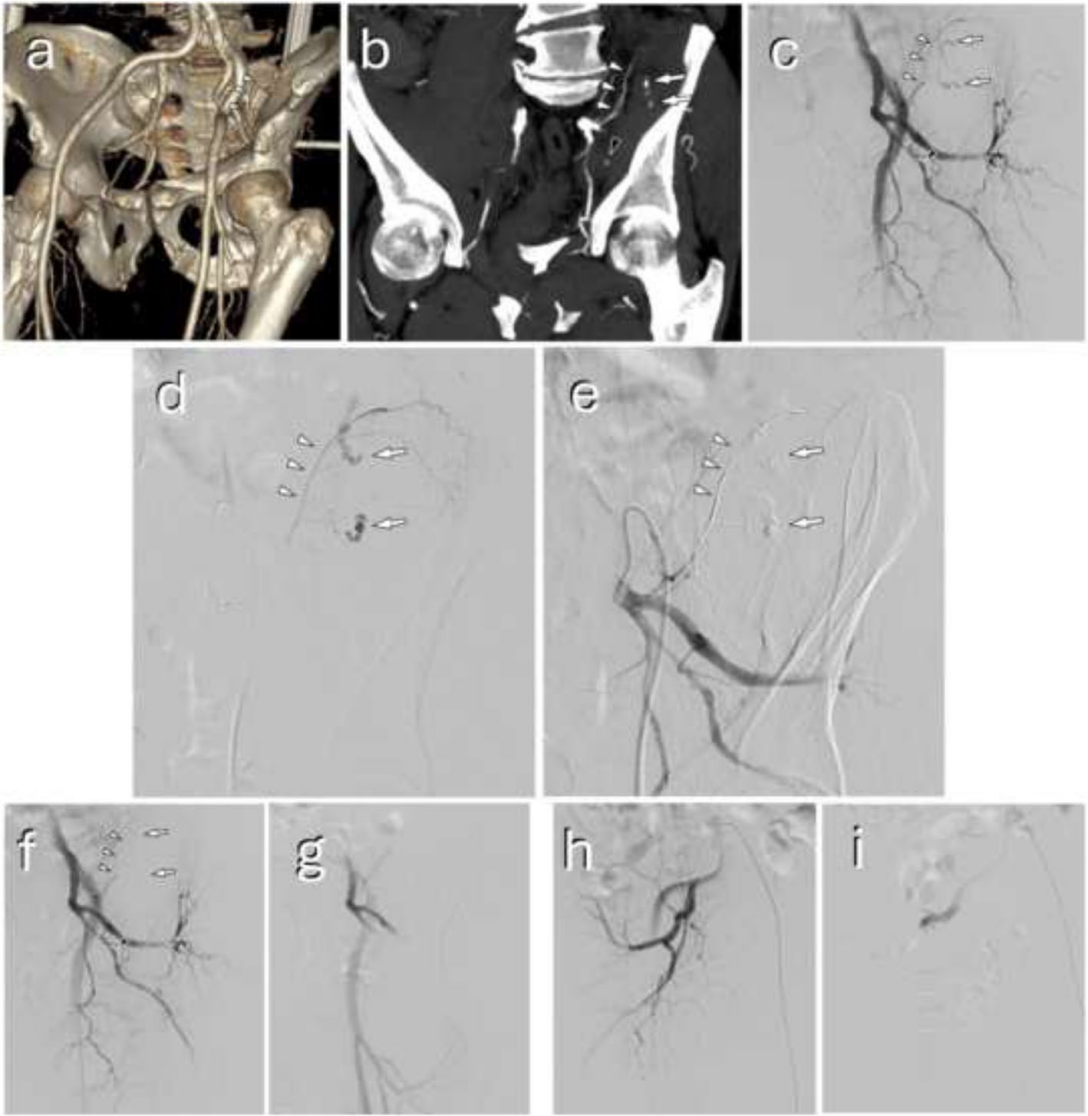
A pelvic trauma managed by selective liquid embolization of active bleeding and bilateral internal iliac resorbable gelatin embolization. A 62-year-old patient presented after a 3-m fall, in a state of hemodynamic instability partially compensated with low-dose vasopressors. a Contrast-enhanced CT revealed a Tile C pelvic fracture. A fragment of the left sacral ala was displaced anteriorly (arrowheads), close to the left internal iliac artery, particularly its posterior trunk branches. b A hematoma was present in this region, along with a markedly irregular iliolumbar artery (white arrowheads) and active bleeding in its vicinity (arrows). Additional smaller-volume active bleeding was also noted (black arrowhead). Following multidisciplinary discussion, interventional radiology management was initiated. c Under local anesthesia, a 6 F ipsilateral catheterization of the left internal iliac artery was performed, and angiography showed two active bleeds (arrows) from the irregular iliolumbar artery (white arrowheads). The pudendal artery appeared clearly dysplastic (black arrowhead), but the active hemorrhage previously visualized in this region had disappeared. d Microcatheterization of the iliolumbar artery with a 2.4-F catheter confirmed the source of bleeding, followed by e complete Onyx-18 (Medtronic, Irvine, CA, USA) embolization (same annotations). f Post-embolization angiography showed complete occlusion of the iliolumbar artery (arrowheads) and contrast extravasation resolution (arrows), with persistent flow through the dysplastic pudendal artery (black arrowhead). g This dysplastic vessel was managed with temporary embolization of the internal iliac artery using resorbable gelatin macrofragments. h No vascular abnormalities were identified on the right side, yet embolization with resorbable gelatin was also performed (i)
